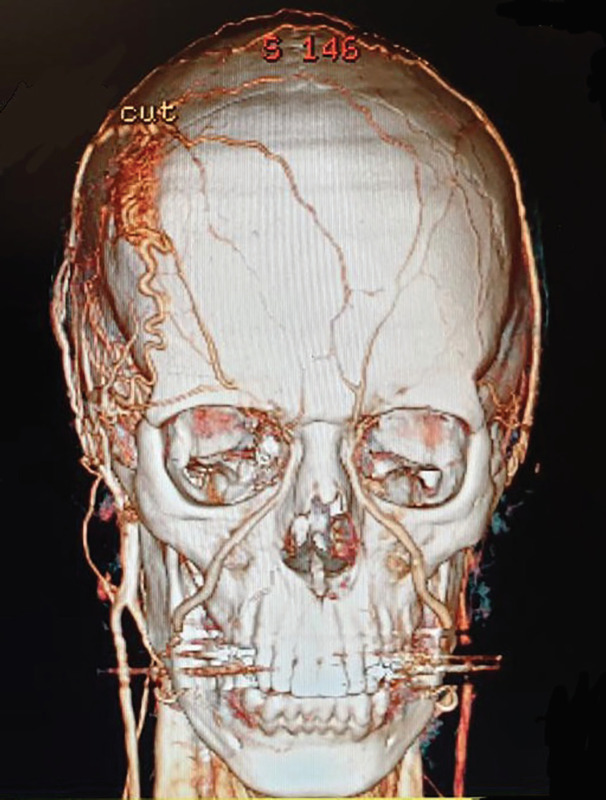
CT angiography revealing arteriovenous malformation in the scalp
